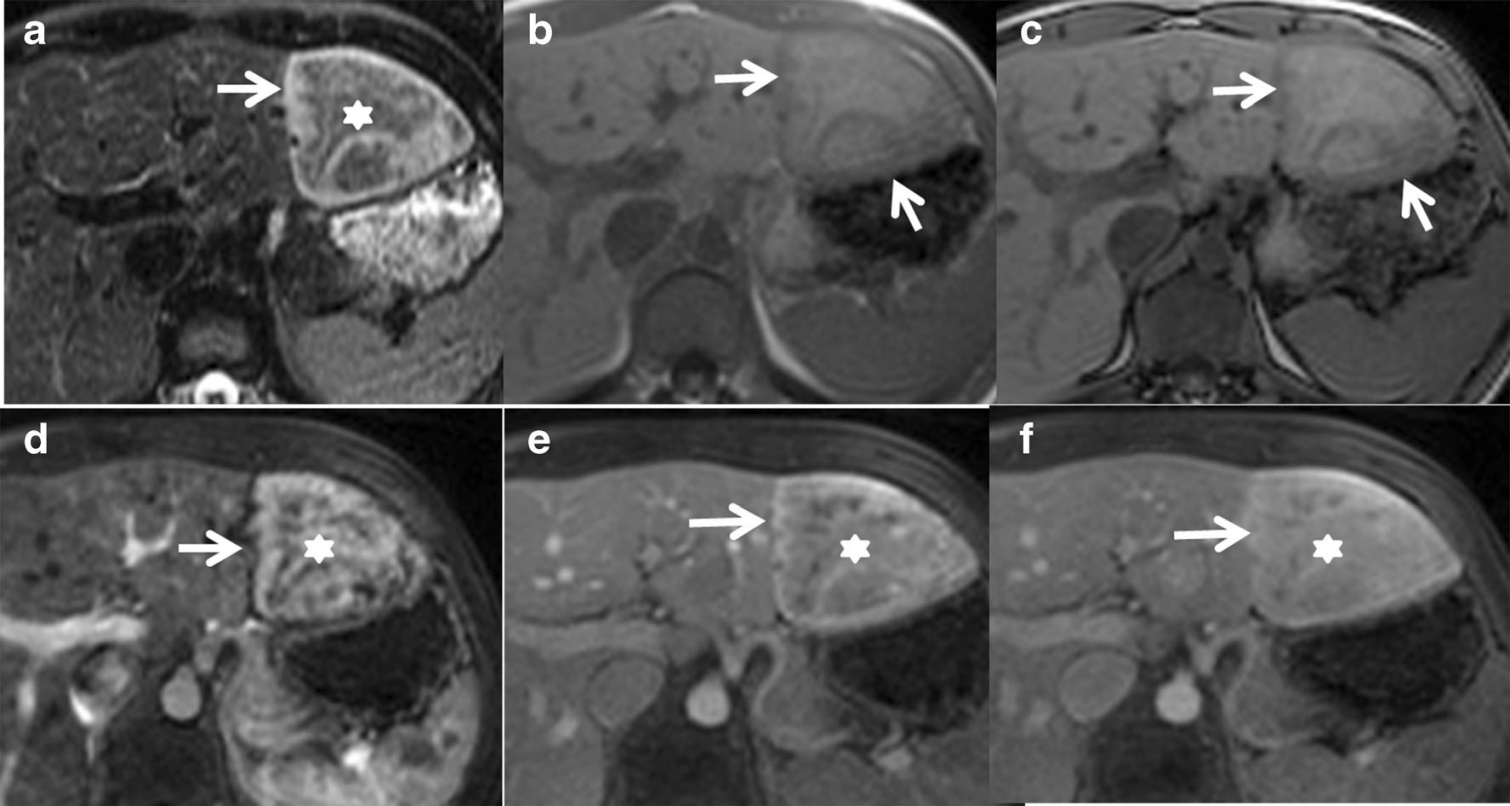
A 30-year-old female with incidental detection of a hypoechoic nodule on ultrasound. (a) Axial fat-saturated T2W MRI shows an iso- to hyperintense lesion (asterisk) in the left lobe of the liver with a peripheral T2 hyperintense rim (*arrow*) representing the atoll sign. Axial T1 in-phase (b) and out-of-phase (c) MRI shows no drop in signal (*arrows*). (d) Axial post-contrast MRI in the arterial phase shows moderate heterogeneous central enhancement (asterisk) and peripheral rim enhancement (*arrow*). Axial post-contrast MRI in the venous phase (e) and delayed phases (f) shows persistent enhancement (asterisk) with delayed enhancement of the peripheral rim (*arrow*). Surgical resection confirmed the diagnosis of inflammatory hepatocellular adenoma
